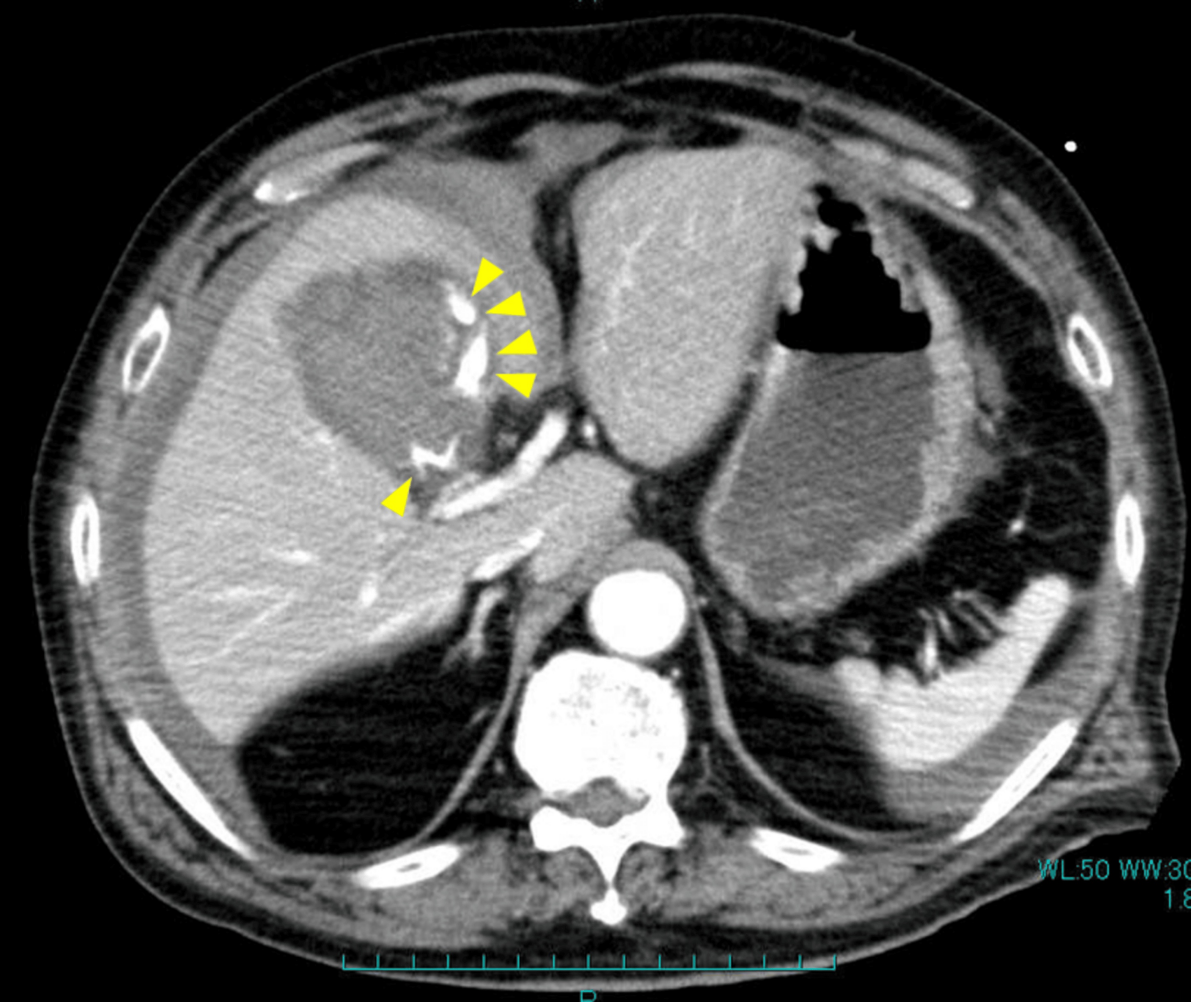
Axial abdominal contrast-enhanced CT scan This image shows gallbladder enlargement with intraluminal hyperdense fluid accumulation and contrast agent extravasation from the cystic artery (arrowheads). Additionally, high-density ascites was observed around the gallbladder, liver, and spleen, raising the suspicion of hemoperitoneum CT: computed tomography
